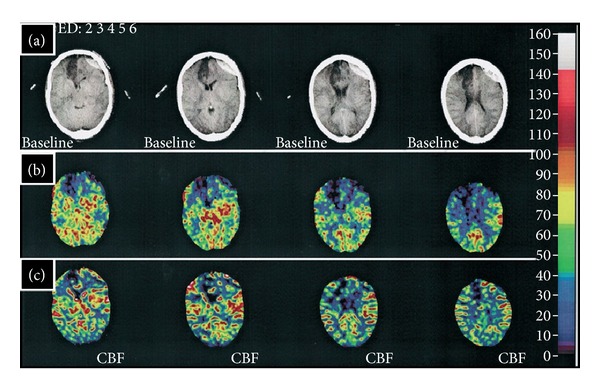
Xenon-Enhanced CT. Baseline CT images (row (a)) indicate a right ACA infarct following aneurysmal rupture of the right ACA. A first Xe/CT study performed with induced hypertension (row (b)) demonstrated a region of ischemia (reduced CBF) in the left frontal region consistent with vasospasm. A second Xe/CT study performed after the withdrawal of pressors (row (c)) did not demonstrate this same region of ischemia. The figure is adapted from [1].
